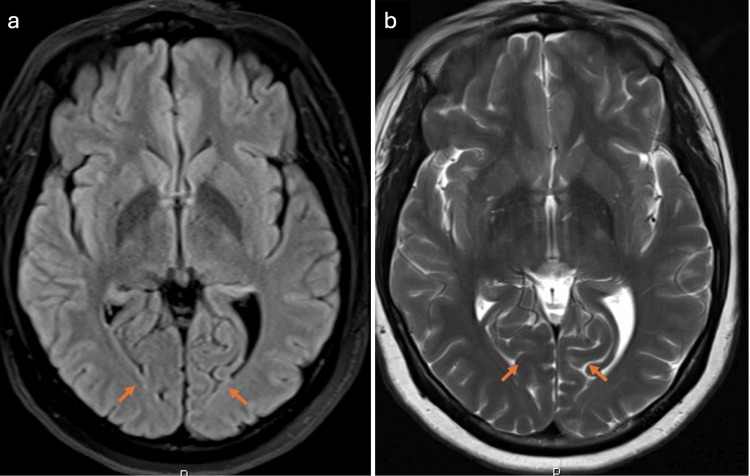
Follow-up brain MRI after three months (a) The bilateral occipito-parietal high signal intensities on FLAIR (arrows) are resolved; (b) T2-weighted imaging sequences (arrows) are resolved FLAIR: Fluid-attenuated inversion recovery
